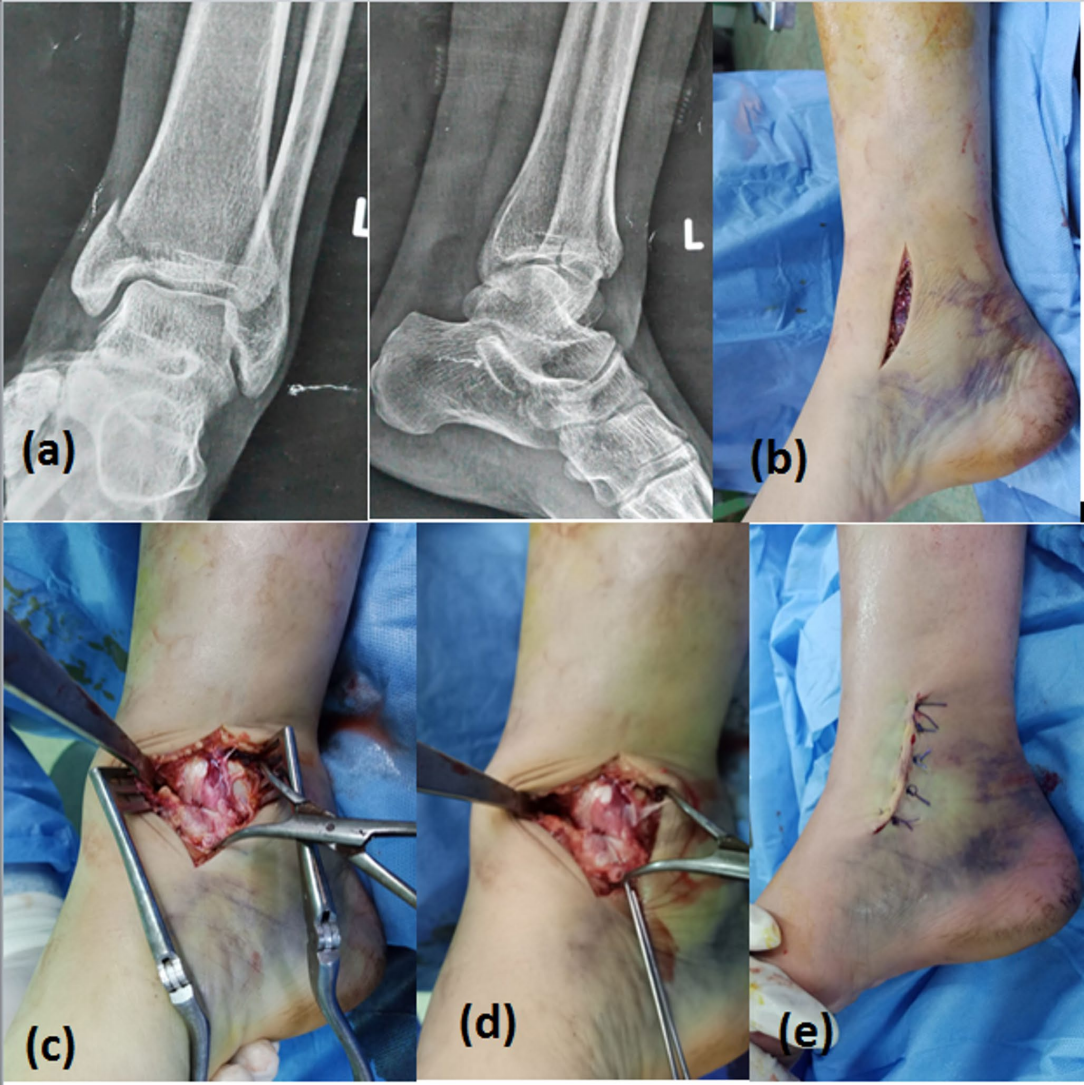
Surgical technique for ORIF: a pre-operative AP and lateral X-ray views of a patient with isolated MM fracture. Intraoperative photograph showing; b the open surgical approach, c pointed forceps holing the reduction, d inserting guide wires for cannulated screws, e skin closure by stitches
